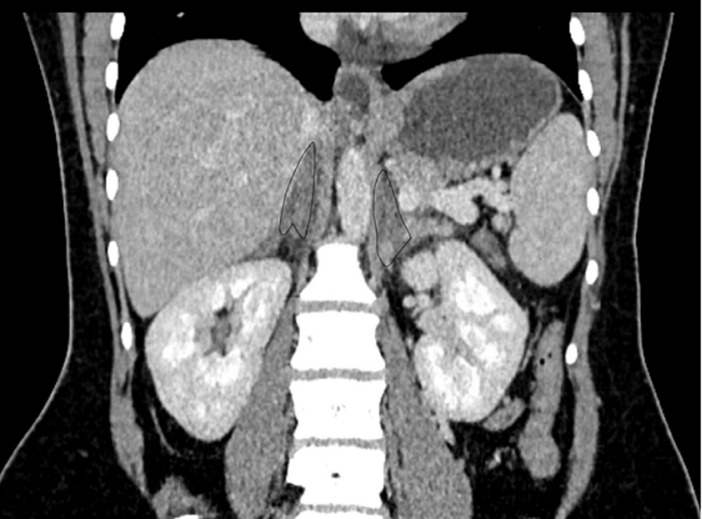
coronal view of contrast-enhanced abdominopelvic computed tomography showing bilateral adrenal gland enlargement with non-enhancing areas in a 34-week pregnant patient
